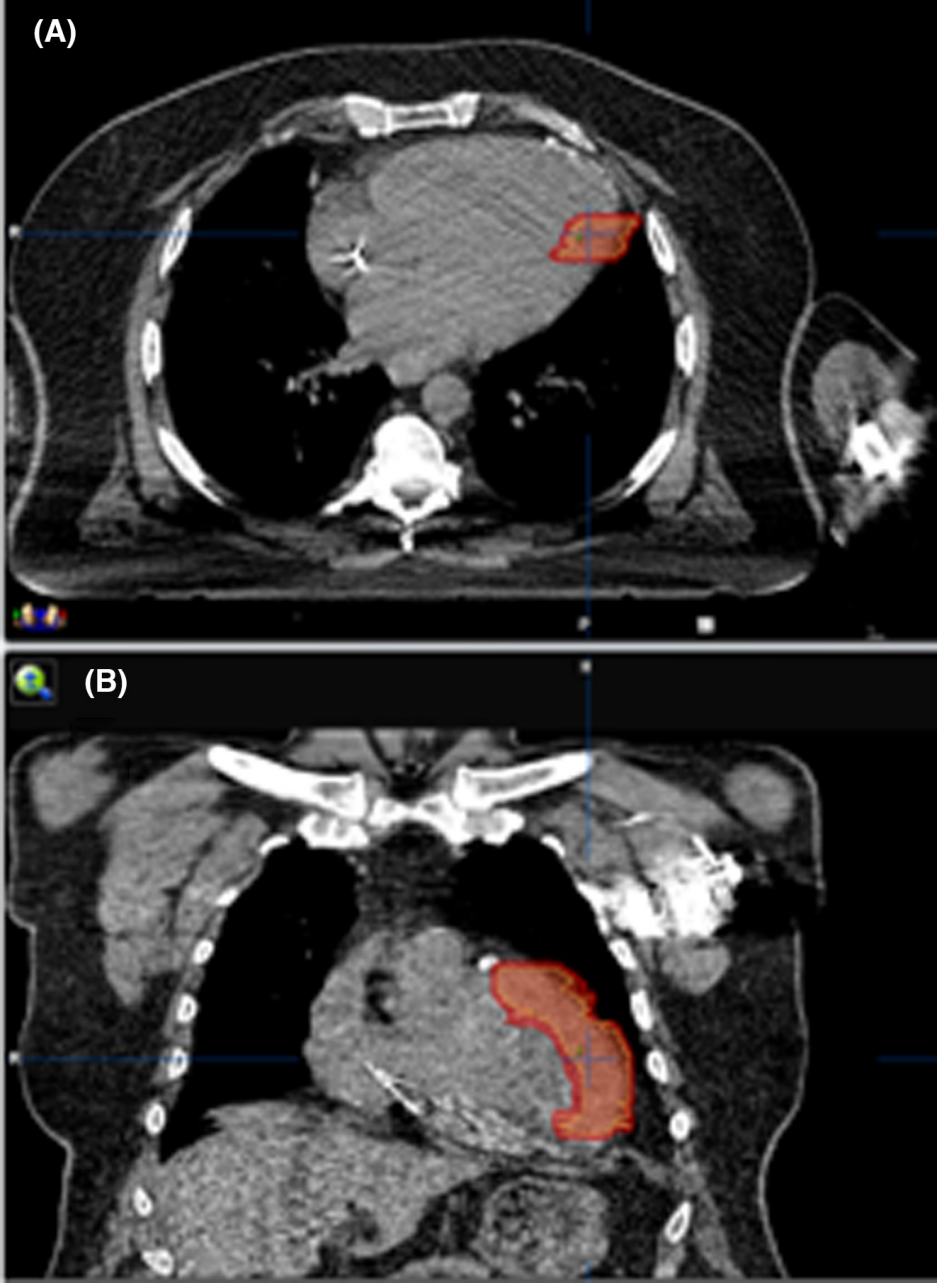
CT scan with the planning target volume highlighted in red. A: axial view. B: coronal view
